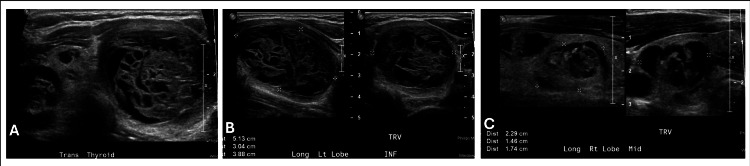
First thyroid ultrasound A. Transverse view of the thyroid gland showing a multinodular goiter; B. Left thyroid lobe nodules, the largest of which measures 5.2 cm; C. Right thyroid nodules, the largest of which measures 2.0 cm
